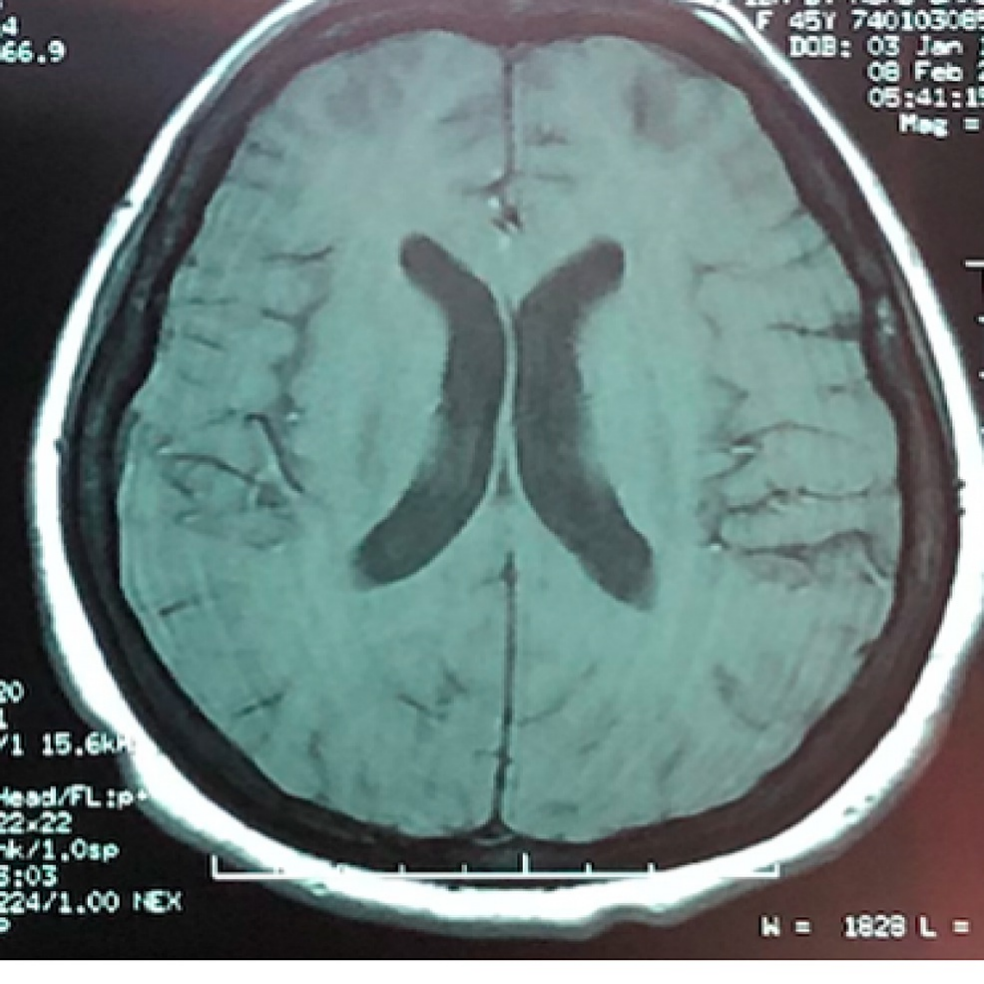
Plain MRI brain showing hydrocephalus.
